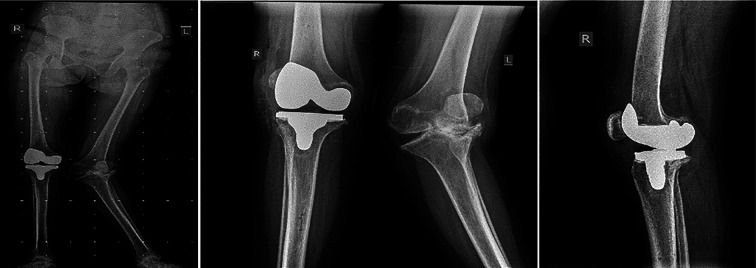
Post-operative X-ray of a unilaterally operated patient at 6 weeks, showing a bilateral lower limb scanogram along with standing anteroposterior and lateral views.
